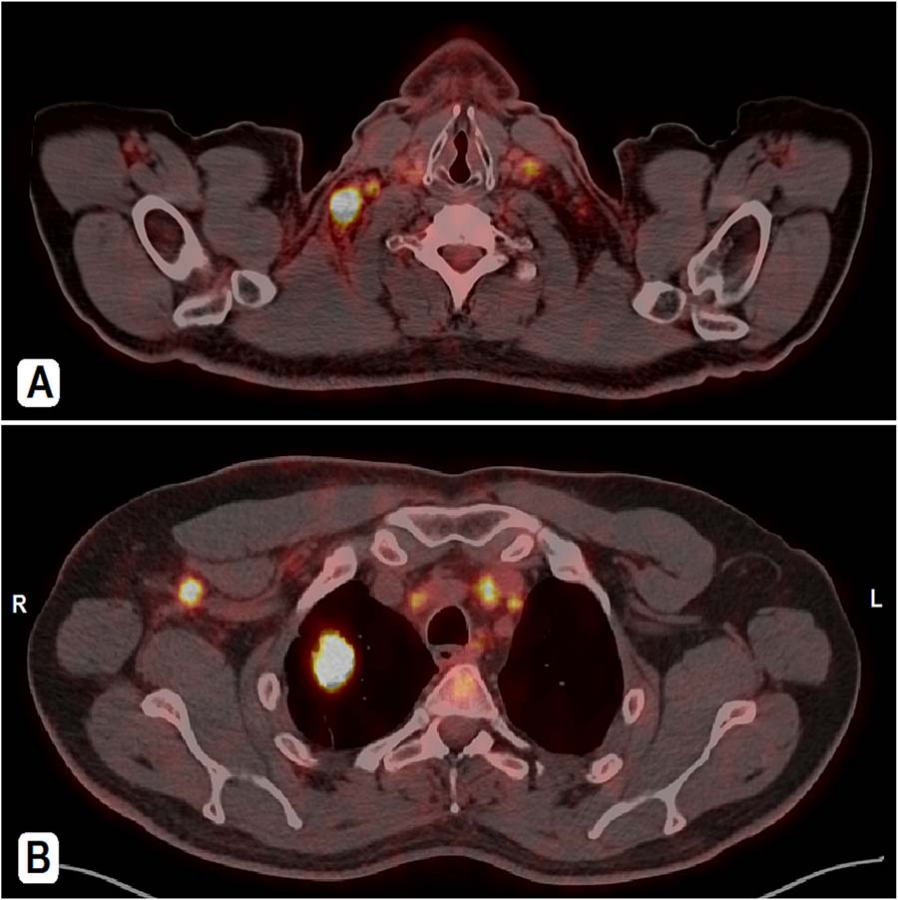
PET-CT scan. (A) At the neck level showing intensely hypermetabolic enlarged cervical lymph nodes. (B) An intensely hypermetabolic right upper lobe lung mass, with metastatic right axillary and mediastinal lymph nodes.
